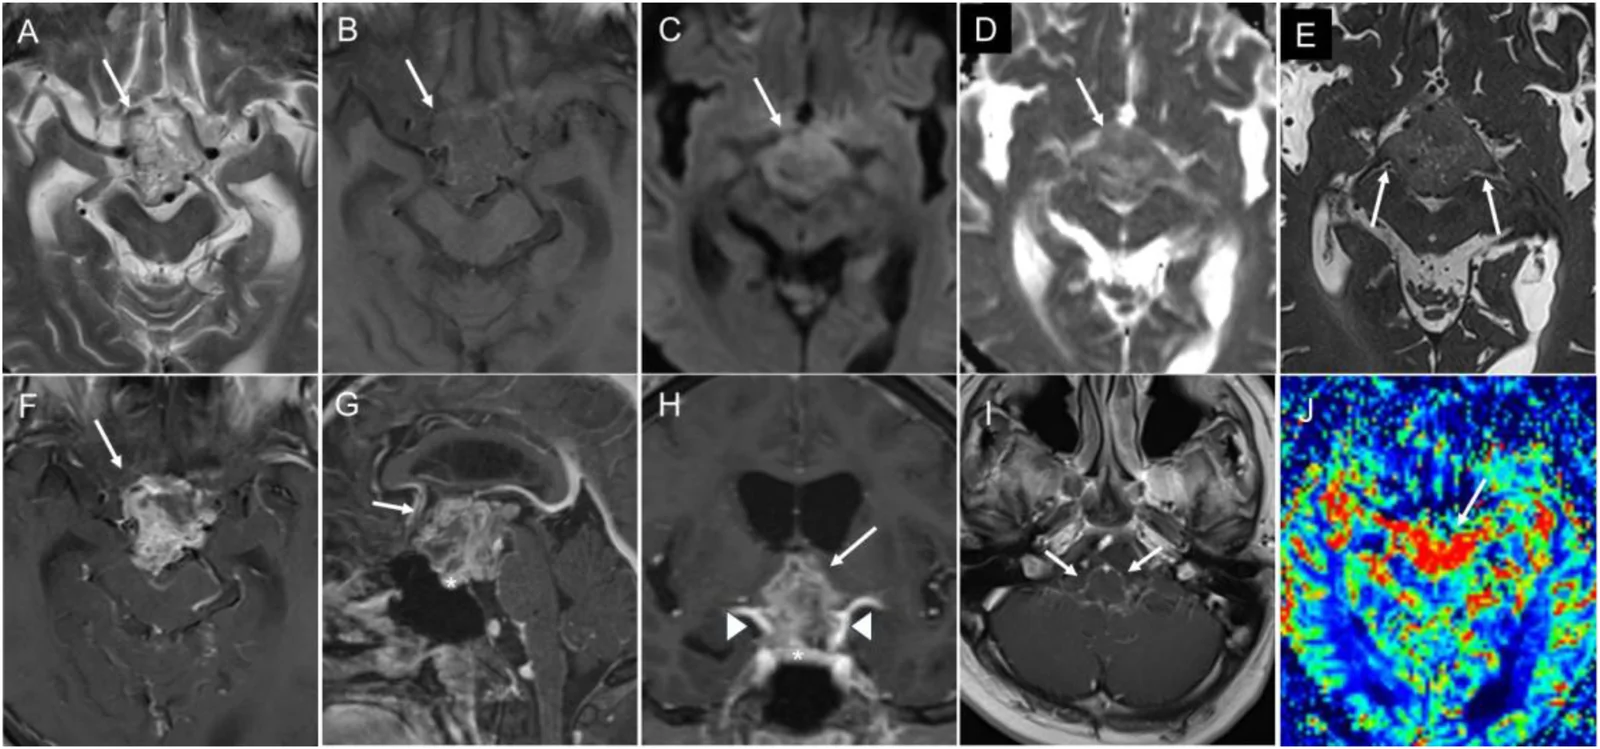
Brain MRI demonstrates a lobulated mass centered in the suprasellar region. The lesion shows heterogeneous hyperintensity with cystic components on T2-weighted images (A, arrow) and heterogeneous hypointensity on T1-weighted images (B, arrow). Diffusion-weighted imaging (C, arrow) shows peripheral hyperintensity with partially decreased ADC values (D); the ADC value is 0.98 × 10^−3^ mm^2^/s (D, arrow). A 3D T2-weighted image (SPACE) demonstrates extension along the optic tracts from the optic chiasm (E, arrows). Contrast-enhanced T1-weighted images (F, axial; G, sagittal; H, coronal) show heterogeneous enhancement with central nonenhancing areas (F-H, arrows). On the coronal image (H), the optic chiasm (arrowhead) is involved by the mass. No abnormalities are observed in the pituitary gland (G and H, *). Leptomeningeal enhancement is observed along the surface of the brainstem (I, arrow). Perfusion imaging shows elevated rCBV (J, arrow). Abbreviations: ADC, apparent diffusion coefficient; rCBV, relative cerebral blood volume; SPACE, sampling perfection with application optimized contrasts using different flip angle evolutions.
